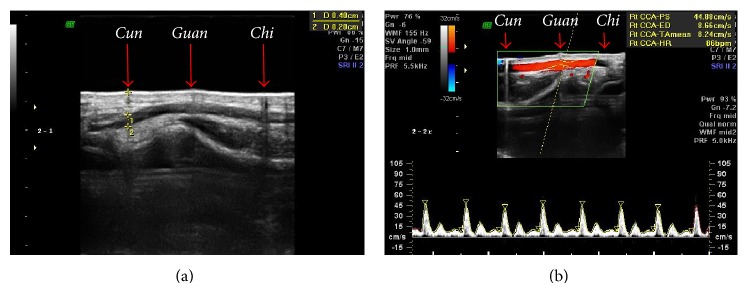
(a) The measurement of the arterial depth and diameter in B-mode and (b) the measurement of the blood flow velocity in the Color Doppler mode.
